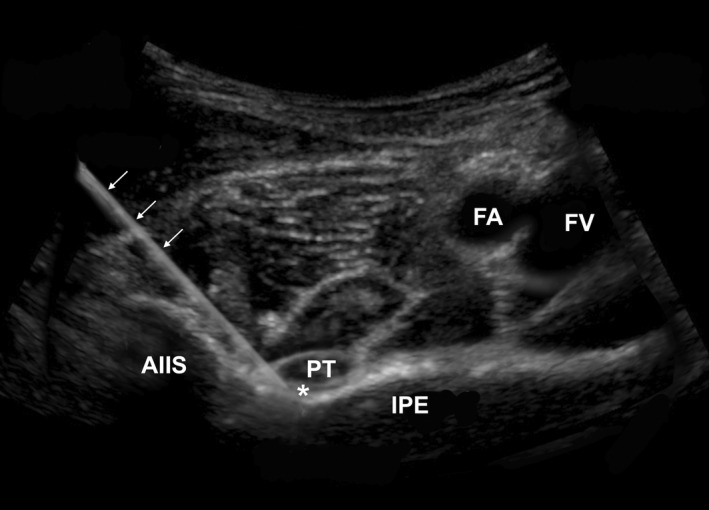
Ultrasound view of the pericapsular nerve group block showing anatomical landmarks, with superimposed needle (arrows) at the target site (*). FA, femoral artery; FV, femoral nerve; AIIS, anterior–inferior iliac spine; PT, psoas tendon; IPE, iliopubic eminence.
